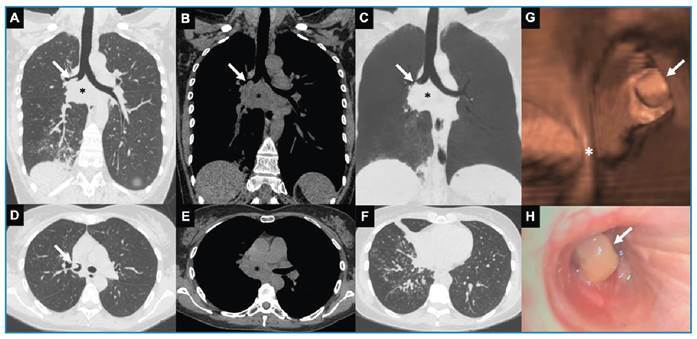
Chest computed tomography (CT) (A-G) and bronchoscopy (H) performed during the investigation of symptoms. (A-C) Coronal oblique images (A: lung window; B: mediastinal window; C: lung window with minimum intensity projection) and (D-F) axial images (D: lung window; E: mediastinal window; F: lung window) display a heterogeneous mass/lymph node conglomerate (black asterisk) with hypodense components suggestive of necrosis in the mediastinum at the subcarinal level, with an endobronchial component (white arrow) extending into the right main and intermediate bronchi related to the middle and right lower lobes. Parenchymal abnormalities including consolidation, centrilobular nodules with a tree-in-bud pattern, and subsegmental atelectasis are present in the middle and right lower lobes. CT virtual bronchoscopy (G) and fiberoptic bronchoscopy (H) reveal an endobronchial lesion with polypoid appearance (white arrow) in the right main/intermediate bronchus (white asterisk: tracheal carina).
